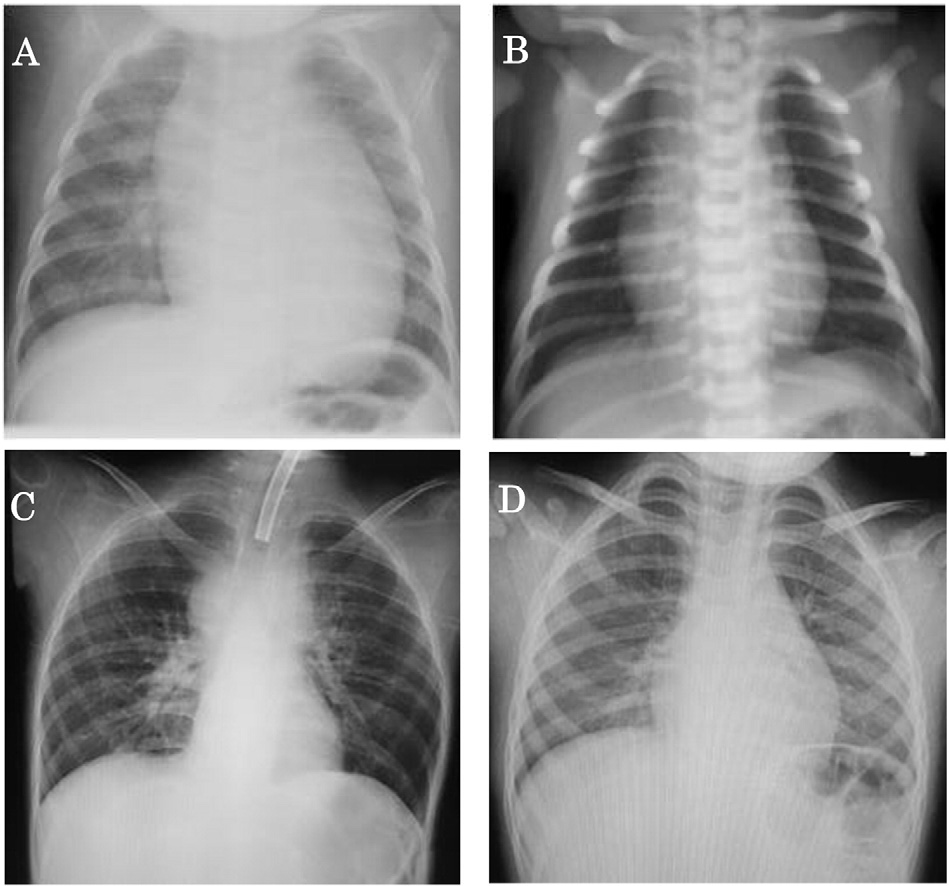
Chest X-ray before and after ERT in two siblings. A. Patient 1 (older sister) at 4 months of age before ERT. Cardiomegaly present (cardiothoracic ratio 65%). B. Patient 2 (younger sister) at birth before ERT. No cardiomegaly present. C. Patient 1 at 7 years of age and after 6 years and 8 months of ERT. No cardiomegaly present. D. Patient 2 at 3 years and 4 months of age and after 3 years and 4 months of ERT. No cardiomegaly present.
